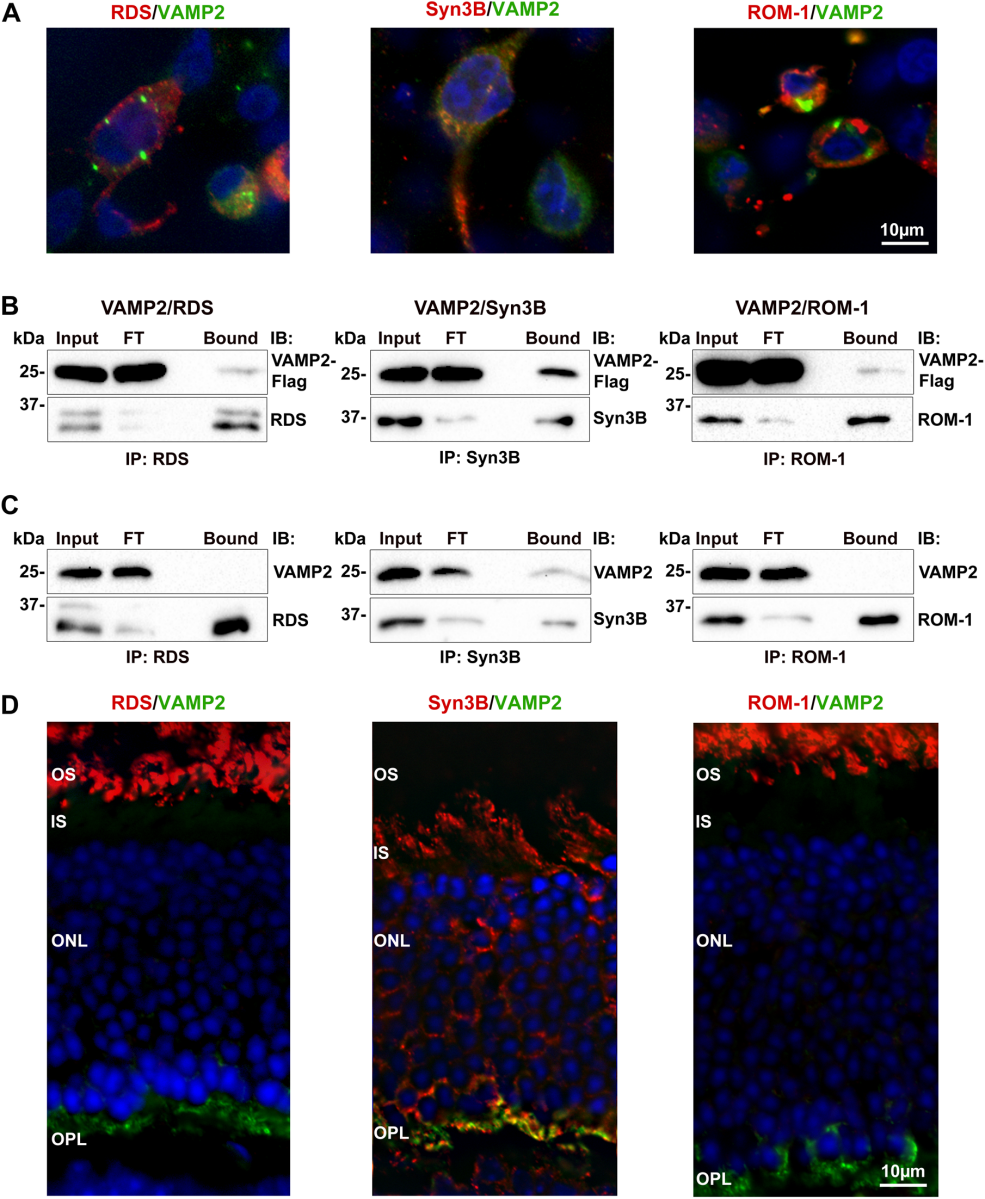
VAMP2 shows a weak interaction with ROM-1 and RDS *in vitro* but not *in vivo*. A. HEK293 cells were co-transfected with vectors containing RDS, ROM-1, or Syn3B and flag-tagged VAMP2 cDNA and fluorescently labeled for RDS (RDS-CT, red) ROM-1 (ROM1-CT, red), Syn3B (Syn3B-529, red), or VAMP2 (anti-DDK, green) antibodies. B. CHAPS-solubilized cell extracts were used for IP with RDS (RDS-CT), ROM-1 (mAB 2H5), and Syn3B (mAB 12E5) antibdoies, and resultant WBs were probed with RDS (mAB 2B7), ROM-1 (mAB 2H5), Syn3B (mAB 12E5), or VAMP2 (anti-DDK) antibodies. C. IP was performed on CHAPS-solubilized WT retinal extracts with the same antibodies as in B, and blots were probed for RDS (mAB 2B7), ROM-1 (mAB 2H5), Syn3B (mAB 12E5), and VAMP2 (VAMP2-SySy) antibodies. D. WT retinal sections underwent IF for VAMP2 (VAMP2-SySy, green), and RDS (RDS-CT, red), ROM-1 (ROM1-CT, red), or Syn3B (Syn3B-529, red) antibodies. Scale bars: 10 μm. OS: outer segment, IS: inner segment, ONL: outer nuclear layer. FT: flow through.
